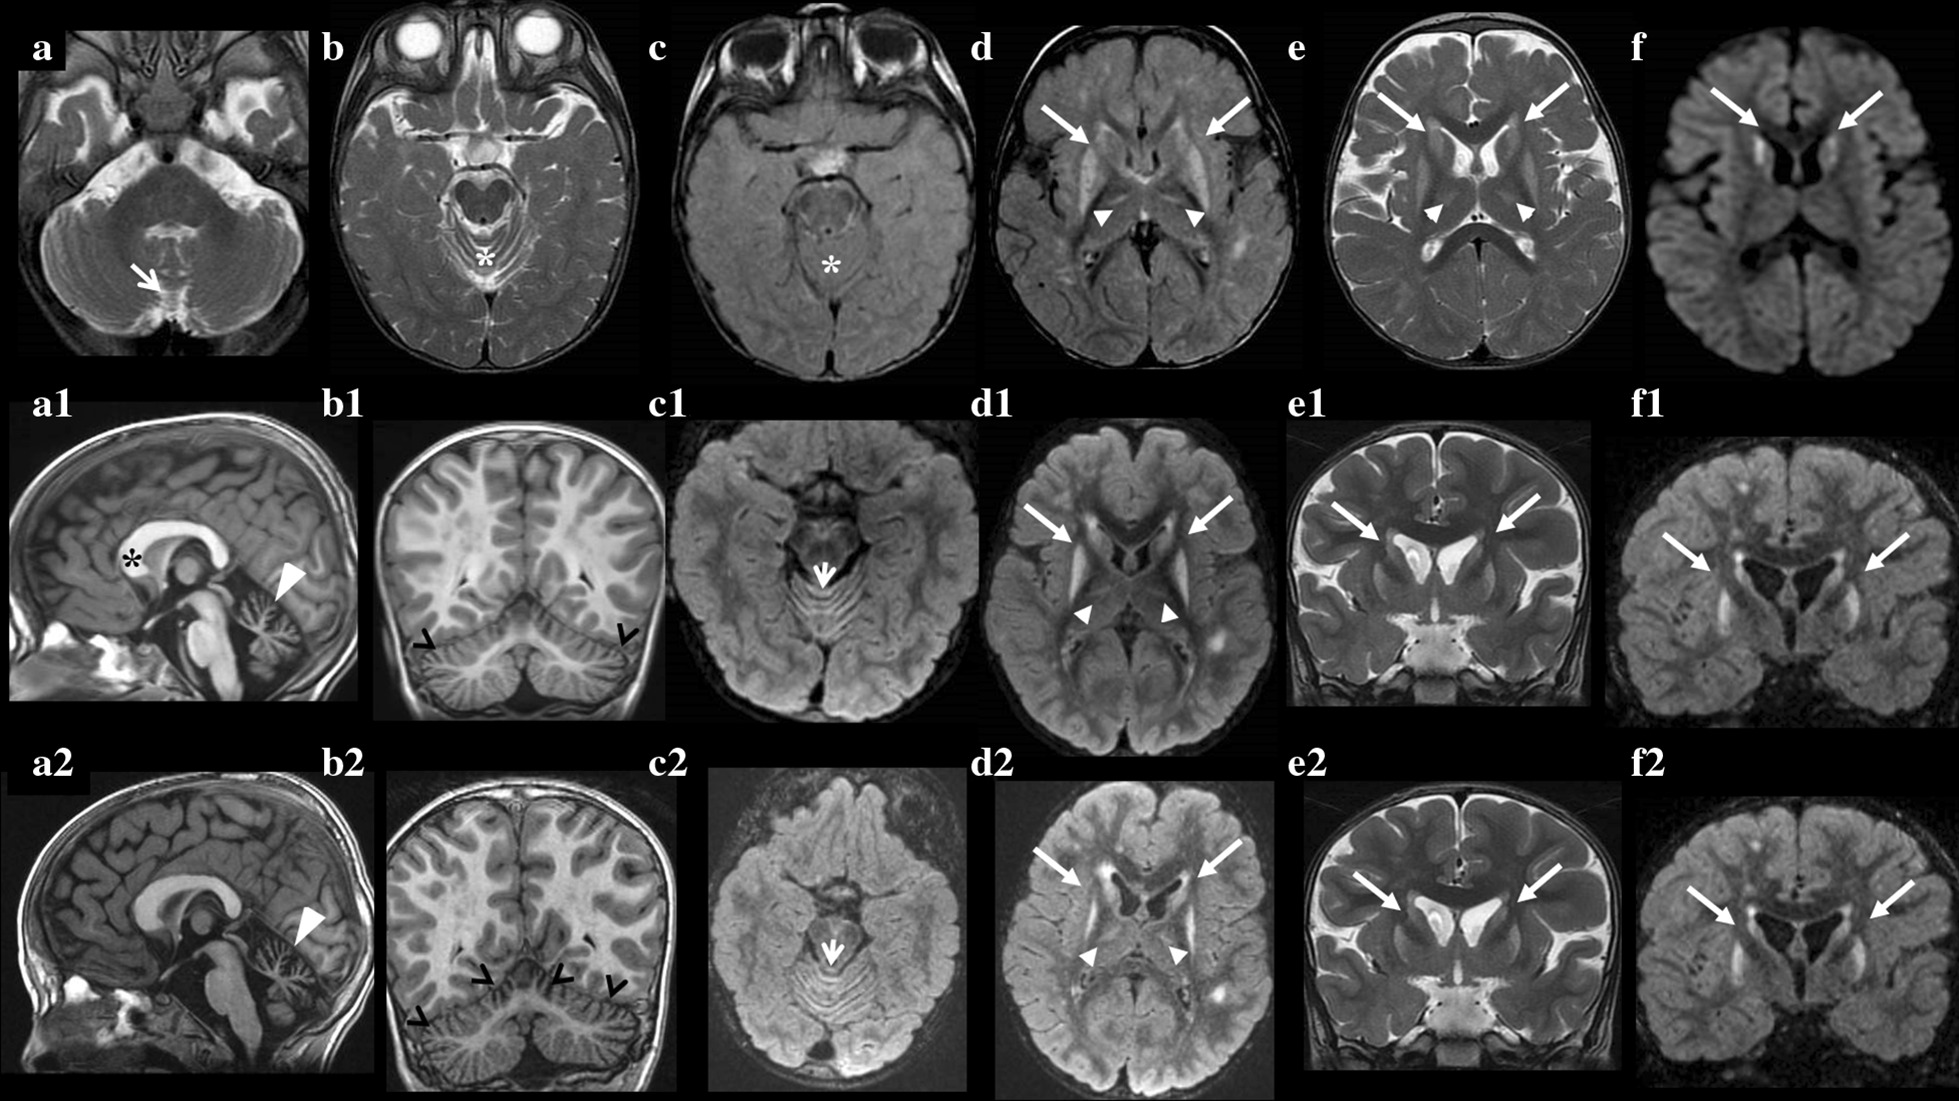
Magnetic Resonance Imaging scans of Patient-1: neuroradiological changes over time. Axial T2 (a, b) and FLAIR-weighted (c) images show slight enlargement of the superior and posterior vermis space (arrow) without signal alteration (asterisks). Axial FLAIR (d) and FSE T2-weighted (e) images show bilateral signal alterations of the striatal nuclei (arrows) and thalami (head-arrows) with reduced diffusivity of the head of the caudate nuclei (arrows) in the diffusion weighted image (f). The second line of MRI images (a1, b1, c1, d1, e1, f1) was performed two years after the first one (a–f). Sagittal (a1) and coronal (b1) T1-weighted images show atrophy of cerebellar vermis, signal alteration of the superior vermis and the initial involvement of cerebellar hemispheres (head-arrows). Sagittal T1-weighted image (a1) also shows a dysmorphic corpus callosum, especially for the hypertrophy of the genu (asterisk). Axial FLAIR-weighted (c1) images show mild cortical hyperintensity of the superior cerebellar vermis. Axial (d1), coronal (f1) FLAIR-weighted and coronal T2-weighted (e1) images show the same signal alterations and lesions in the supratentorial region of the first line ones (a–f), except for a slight reduction in the signal alteration of striatal nuclei, which also appear more swollen (arrows) (d1). A lack of restriction of proton diffusivity is also evident. The third line of MRI images (a2, b2, c2, d2, e2, f2) was performed 2 years after the second one (a1, b1, c1, d1, e1, f1). Sagittal (a2) and coronal (b2) T1-weighted images show the progression of cerebellar atrophy (head-arrows). An increased signal alteration of the superior vermis is evident in axial FLAIR-weighted image (arrow) (c2). (d2, e2, f2) images, compared to the same sequence images in the second line (d1, e1, f1) show no new signal alteration or lesion of the supratentorial region
